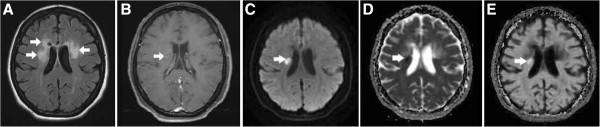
A type IA lesion in a patient with MS. (A) Axial T2-FLAIR brain MRI shows several demyelinating lesions in the bilateral periventricular white matter (arrows). (B) CE T1WI shows a small central nodular enhancement in one of the lesions on the right side (arrow). (C–E) The lesion (arrow) is hyperintense on the DWI, hypointense on the ADC map, and hyperintense on the eADC map, indicating an early stage of inflammation with restricted water diffusion (cytotoxic edema).
